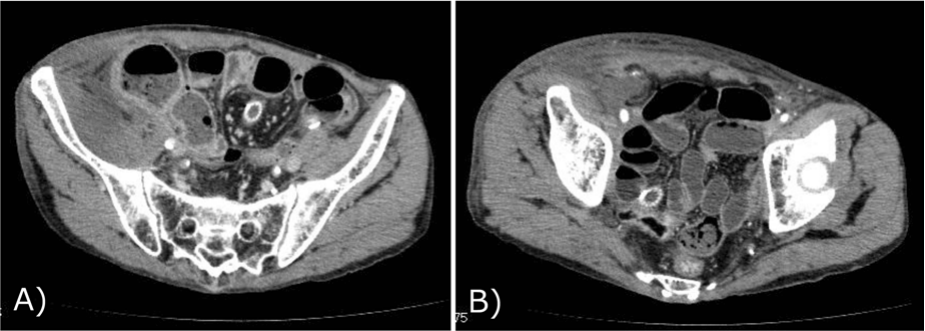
CT findings before the debridement; A) showed the retroperitoneal abscess. B) showed the normal external iliac artery.
